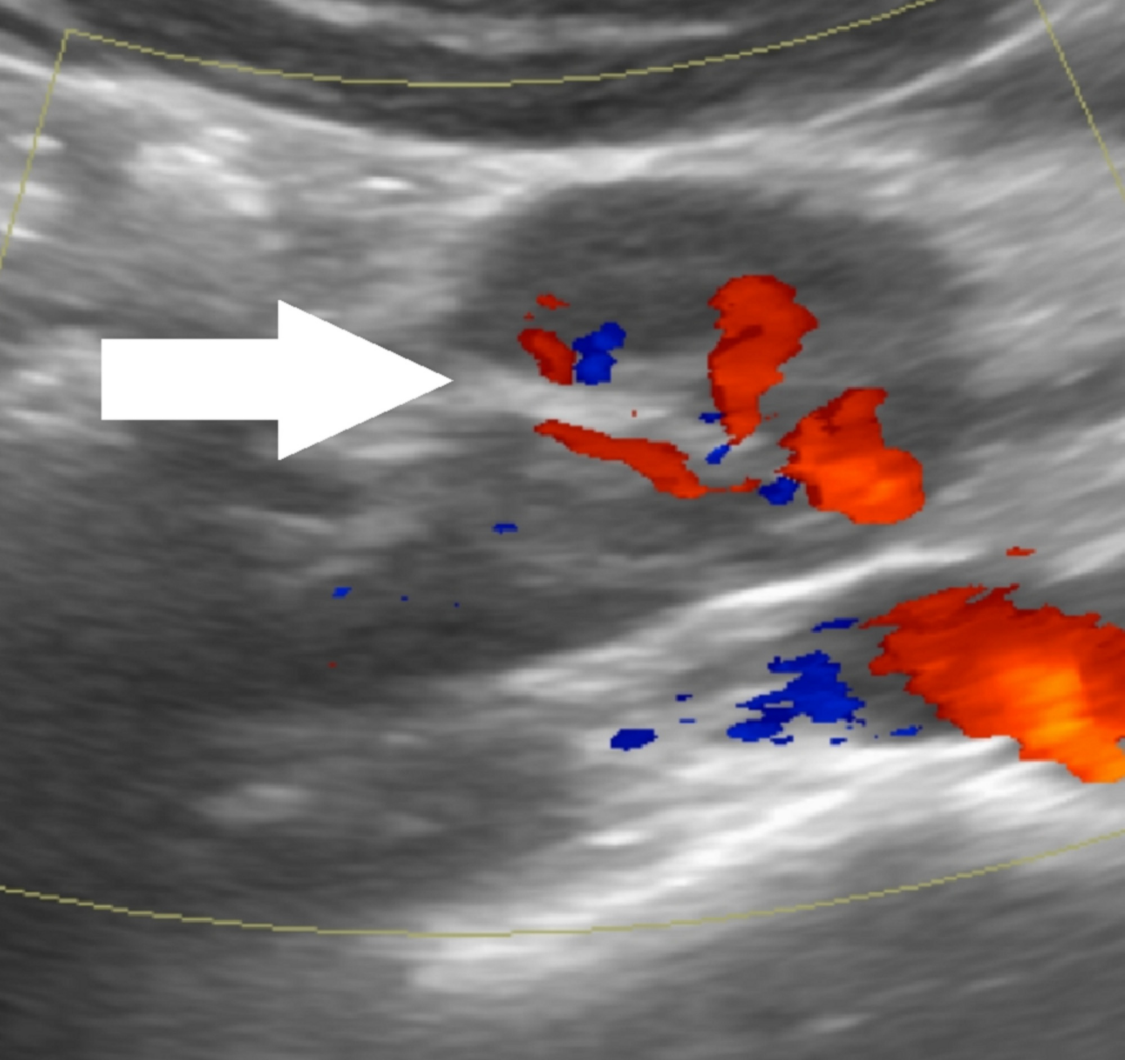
Doppler Ultrasound Image Demonstrating a Vascular Pedicle Doppler ultrasound image demonstrating the reniform appearance of the mass with a central vascular pedicle.
